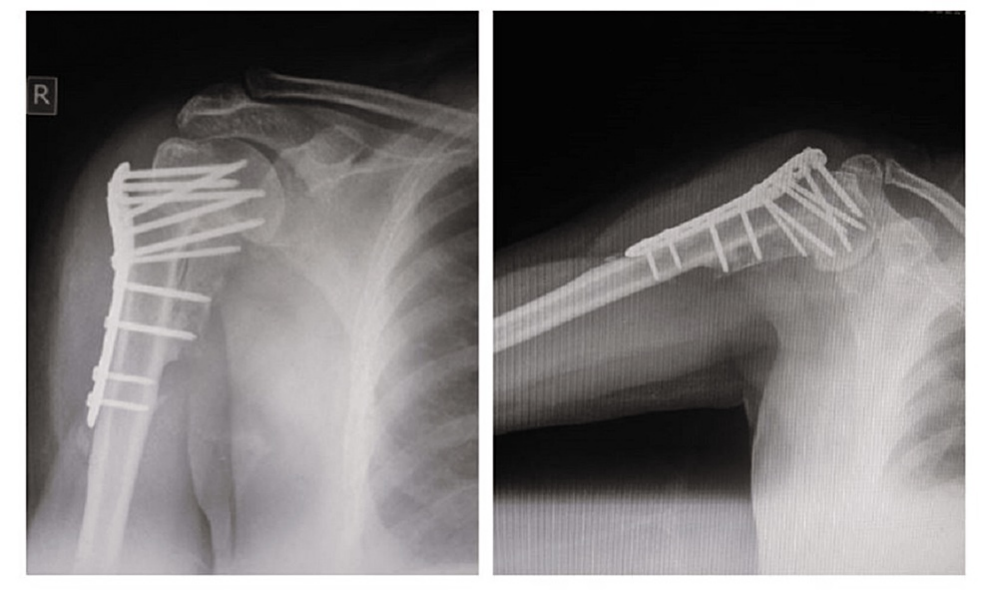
Post-operative X-ray showing union and callus.
